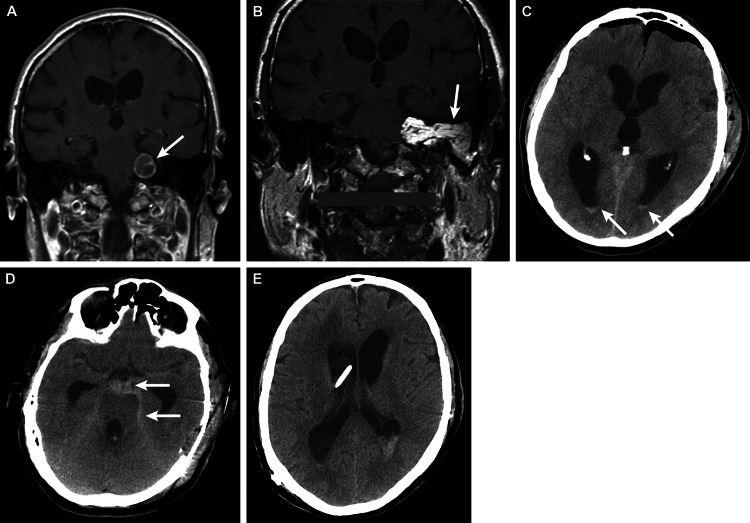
Case 1 – a 68-year-old man who presented with left-sided hearing loss, vertigo, and significant left-sided facial weakness (A) Preoperative coronal T1-weighted brain MRI displayed a left cerebellopontine (CP) angle-enhancing lesion (arrow), suggestive of a vestibular schwannoma, which measured approximately 1.7 × 1.7 cm, with no evident midbrain shift. (B) Postoperative coronal T1-weighted MRI showed expected postoperative changes from a left translabyrinthine craniotomy for resection. Fat packing could be visualized within the left CP angle and left mastoid air cells (arrow). (C and D) Axial CT on postoperative day two showed postoperative changes with no evidence of midline shift. (C) An intraventricular hemorrhage was revealed, and blood products could be seen in the occipital horns of lateral ventricles (white arrows), in addition to ventriculomegaly, indicating hydrocephalus. (D) A new perimesencephalic subarachnoid hemorrhage that extended to the suprasellar cisterns (arrows) could be detected. (E) Axial CT showing external ventricular drain placement in the frontal horn of the right lateral ventricle following intracerebral hemorrhage MRI: magnetic resonance imaging; CT: computed tomography Used with permission from Barrow Neurological Institute, Phoenix, Arizona
